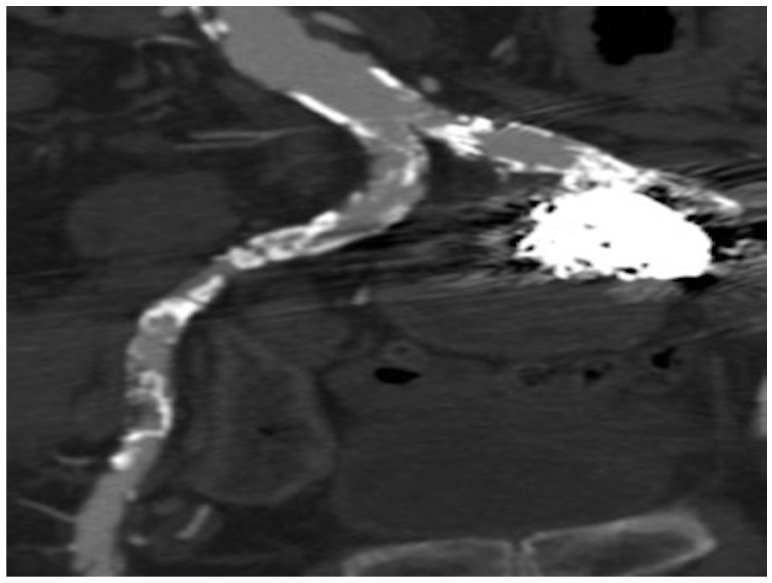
Post-embolization follow-up coronal CTA with contrast demonstrated complete closure of the pseudoaneurysm.
